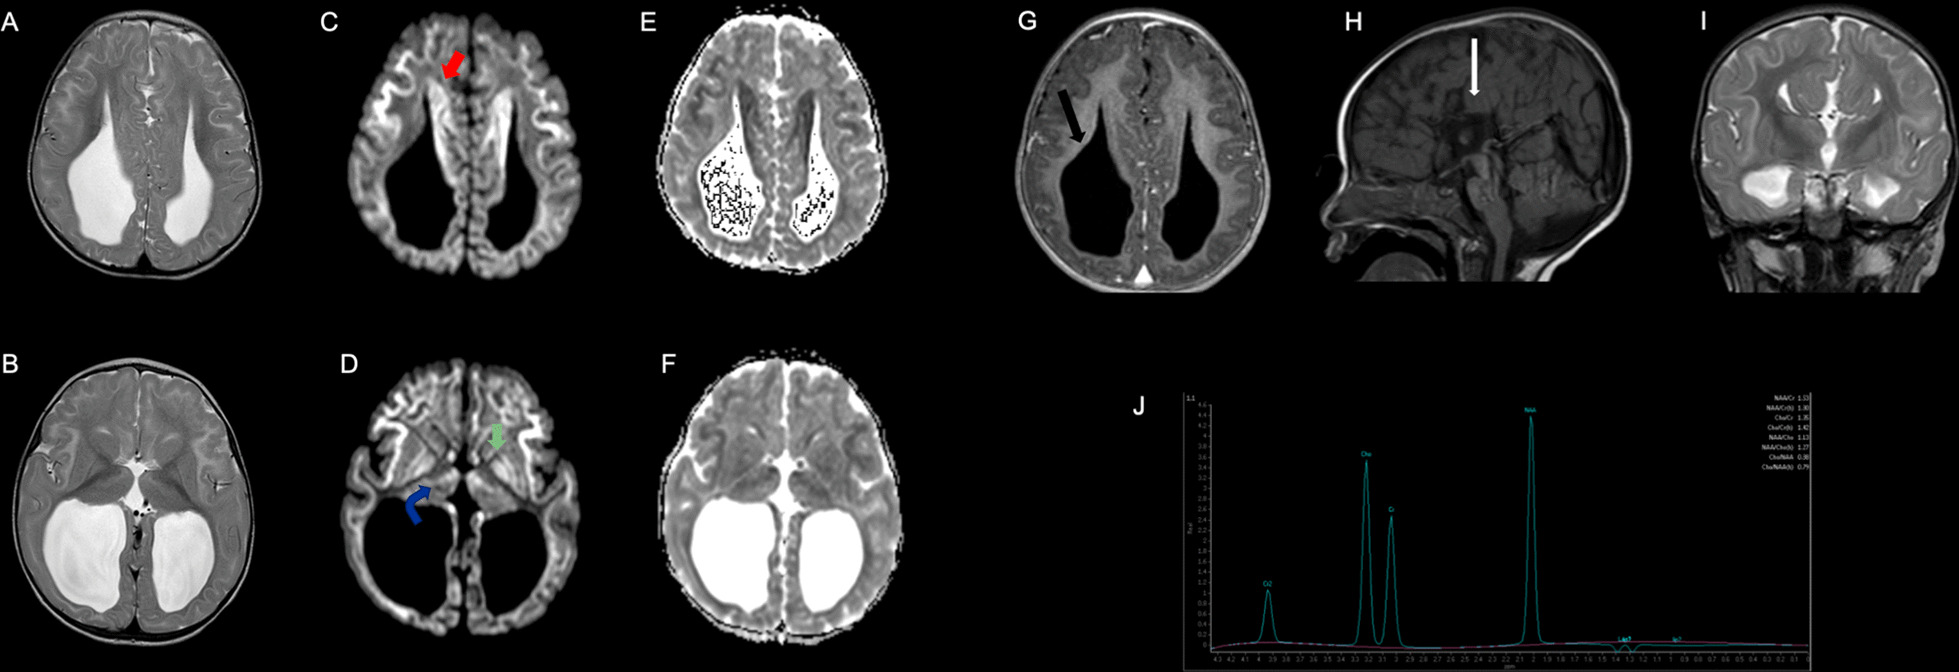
Brain MRI of the present patient at 23 months of age, showing extensive bilateral symmetric, anomalous signals for the subcortical white matter and basal ganglia, and corpus callosum agenesia
